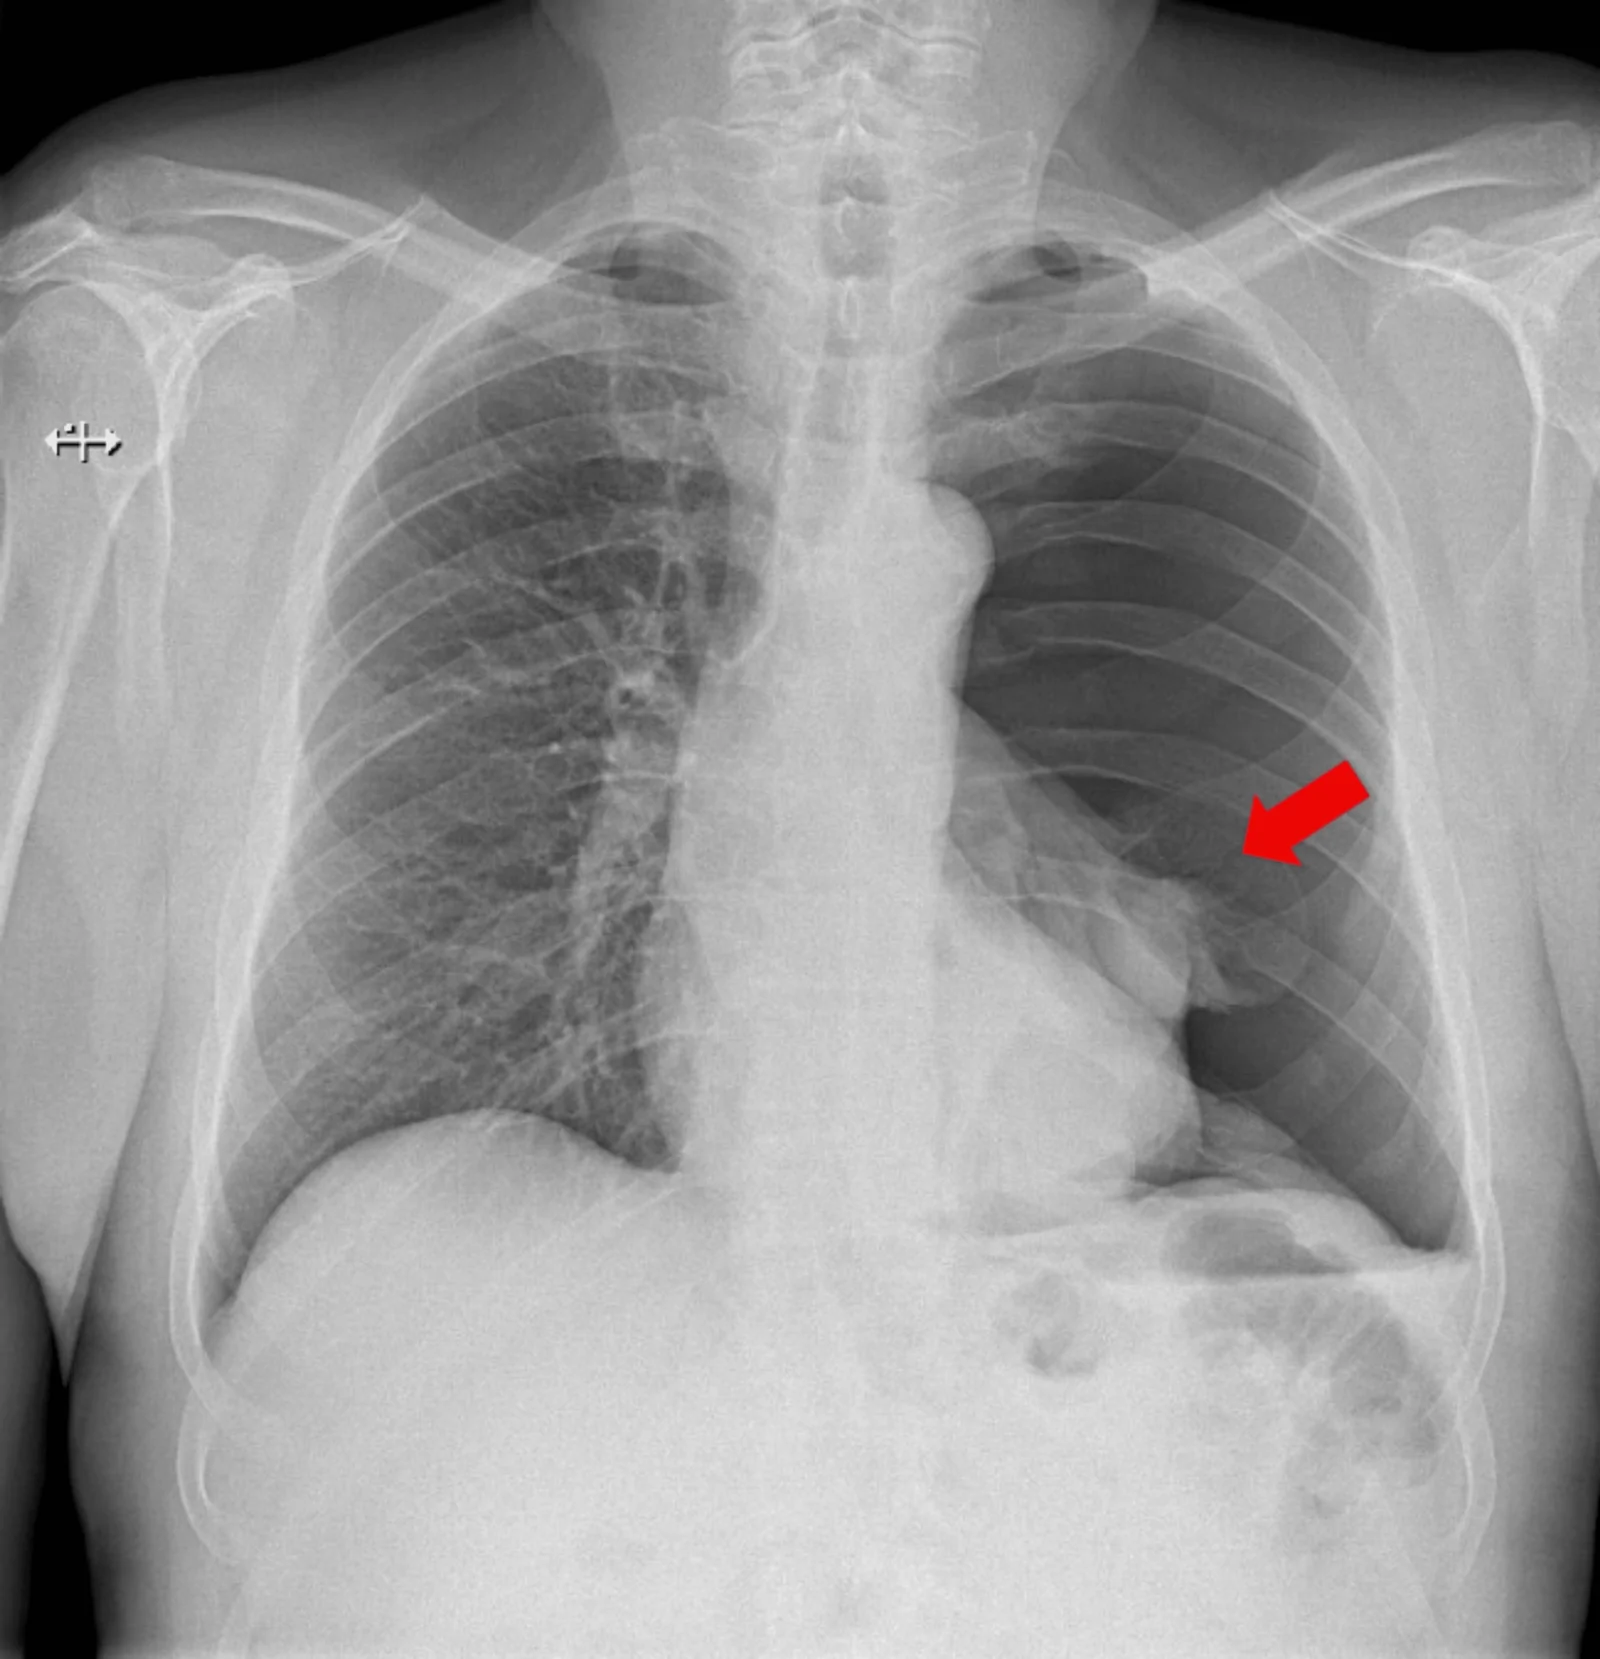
Chest radiograph obtained one week later demonstrating recurrent collapse of the left lung. The red arrow indicates the recurrently collapsed left lung.
